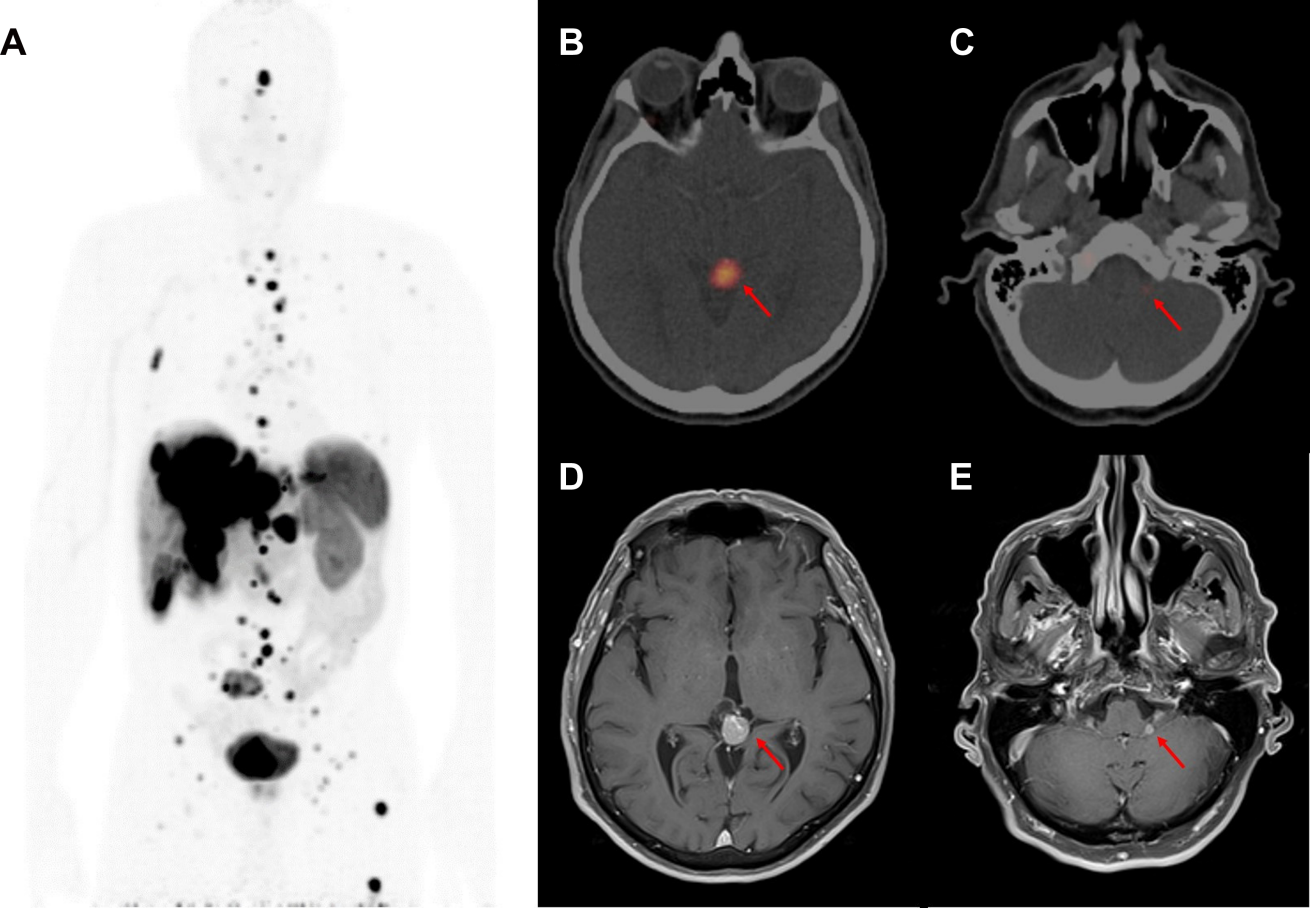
Initial diagnosis of central nervous system metastases at 34 months after diagnosis. (A) Inverted maximal intensity projection on coronal view from the ^68^Ga-dotatate PET/CT performed at 34 months after diagnosis showing the extent of metastatic disease expressing somatostatin receptors. (B) ^68^Ga-dotatate PET/CT showing an avid lesion in the pineal gland (SUVmax 36.4) measuring 1.3 cm × 1.1 cm. (C) ^68^Ga-dotatate PET/CT showing a small avid left cerebellopontine angle lesion (SUVmax 7.0). (D) MRI brain T1-post-gadolinium sequence performed at 35 months after diagnosis showing a 1.6 cm × 1.5 cm enhancing pineal mass. (E) MRI brain T1-post-gadolinium sequence showing a 0.5 cm × 0.4 cm left cerebellopontine angle enhancing lesion.
